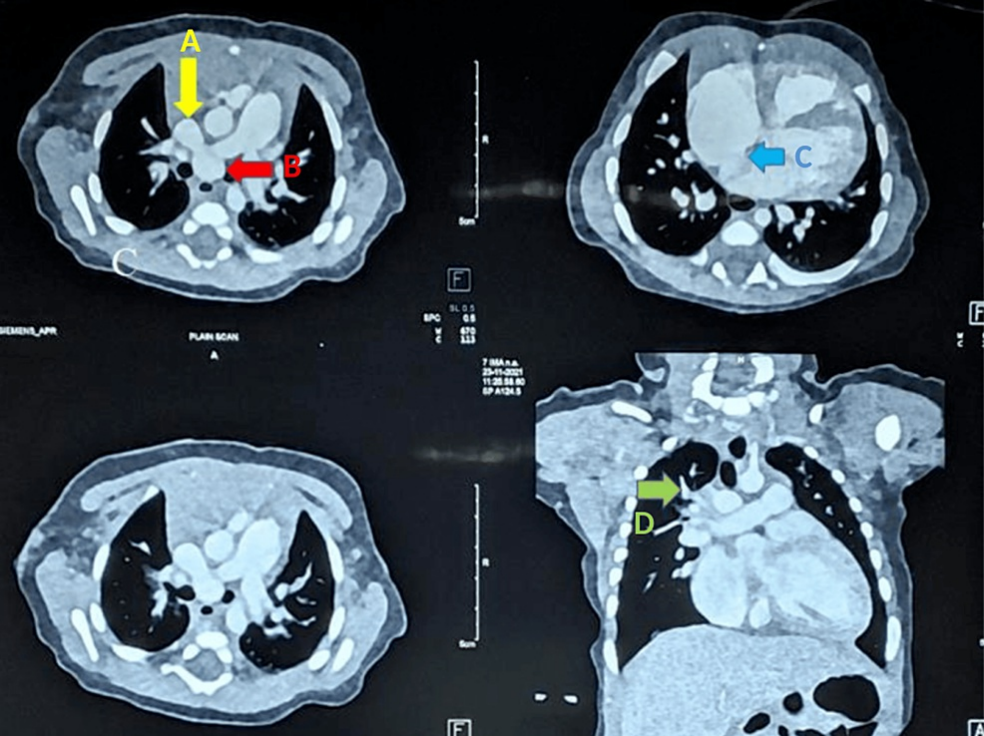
Contrast-enhanced CT angiography thorax in axial section showing common tubular vascular channel (arrow A) draining into superior vena cava (arrow B). Arrow C shows dilated right atrium and right ventricle with a small atrial septal defect. Arrow D shows pulmonary veins draining into the common tubular vascular channel. Overall findings are suggestive of supracardiac total anomalous pulmonary venous drainage with the atrial septal defect.
